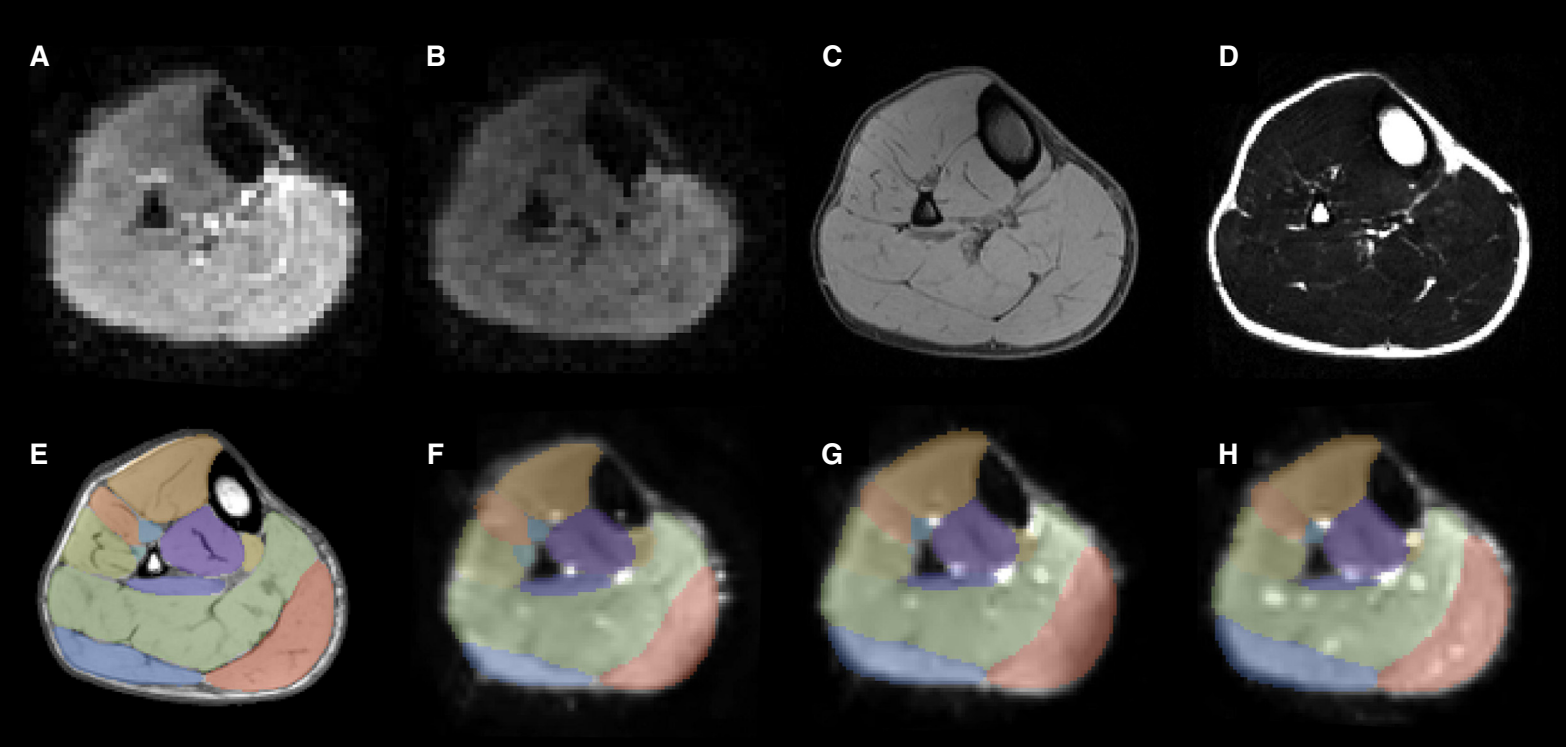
Transverse slice in a volunteer: raw MRI data and muscle segmentation. (A) DT‐MRI image obtained with b = 0 sec/mm^2^. (B) DT‐MRI image obtained with b = 400 sec/mm^2^. (C) Water image from the mDixon scan. (D) Fat image from the mDixon scan. (E) Muscle segmentation overlaid to the out‐of‐phase mDixon image. (F, G, H) Muscle segmentation obtained using the down‐sampled out‐of‐phase mDixon images overlaid to the up‐scaled diffusion data. (F) 15° dorsiflexion; (G) Neutral position; (H) 30° plantarflexion.
